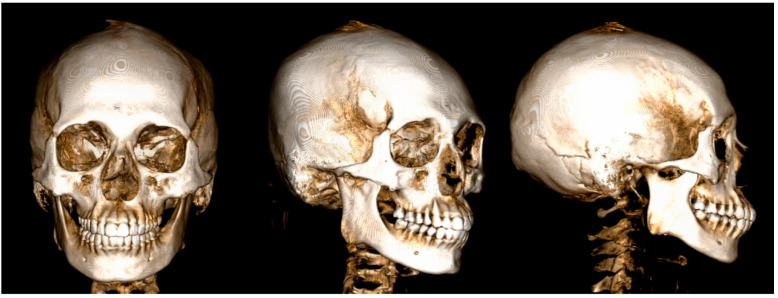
Three-dimensional views of the computed tomography data of the patient.
